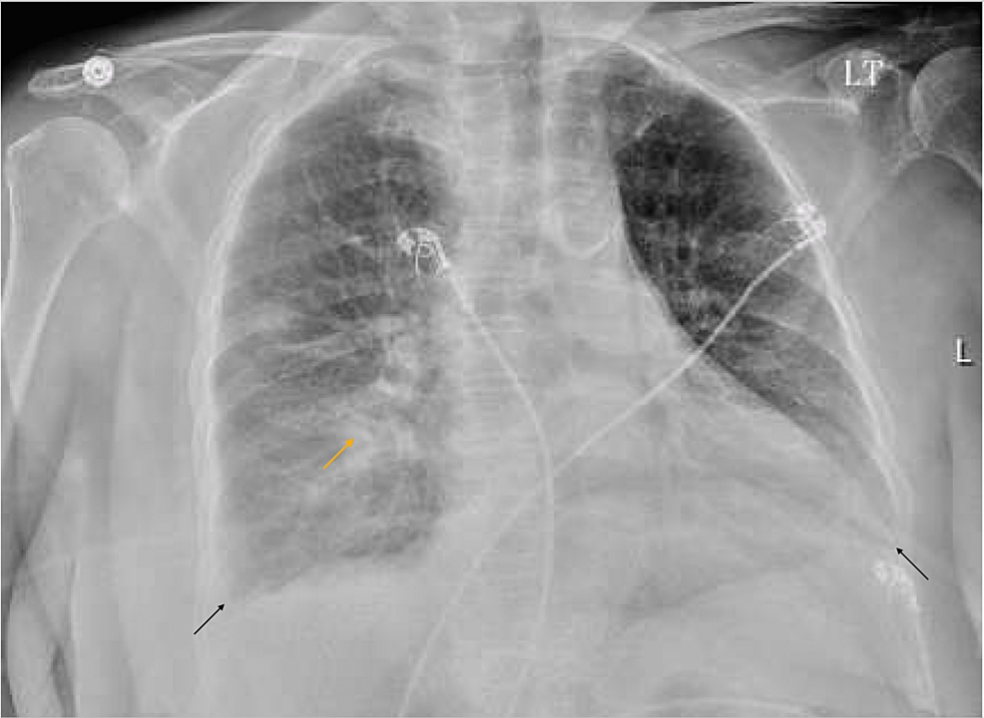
Chest X-ray on admission Mild bilateral pleural effusion (black arrow) and consolidation versus lymphadenopathy on right midlung and basilar airspace (yellow arrow).
